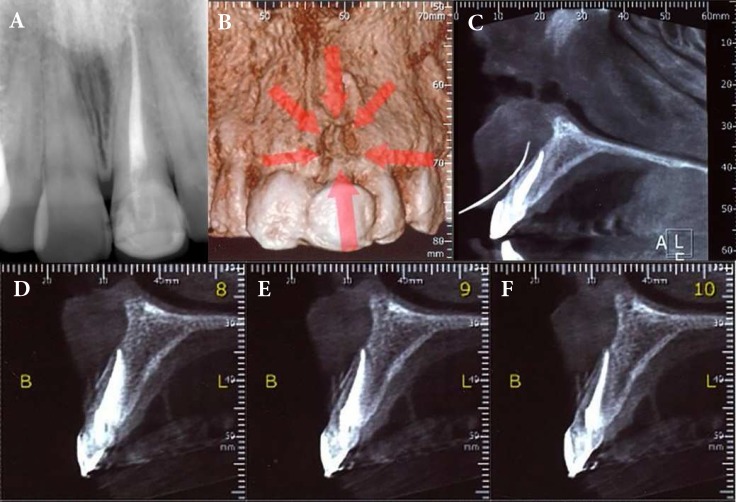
A)* Periapical radiography shows flawless RCT; *B)* Three-dimensional reconstruction provided by the CBCT software showing a bony lesion; *C)* Deviation of the tracing gutta-percha cone to the labial soft tissues; *D-F)* Normal CBCT findings
